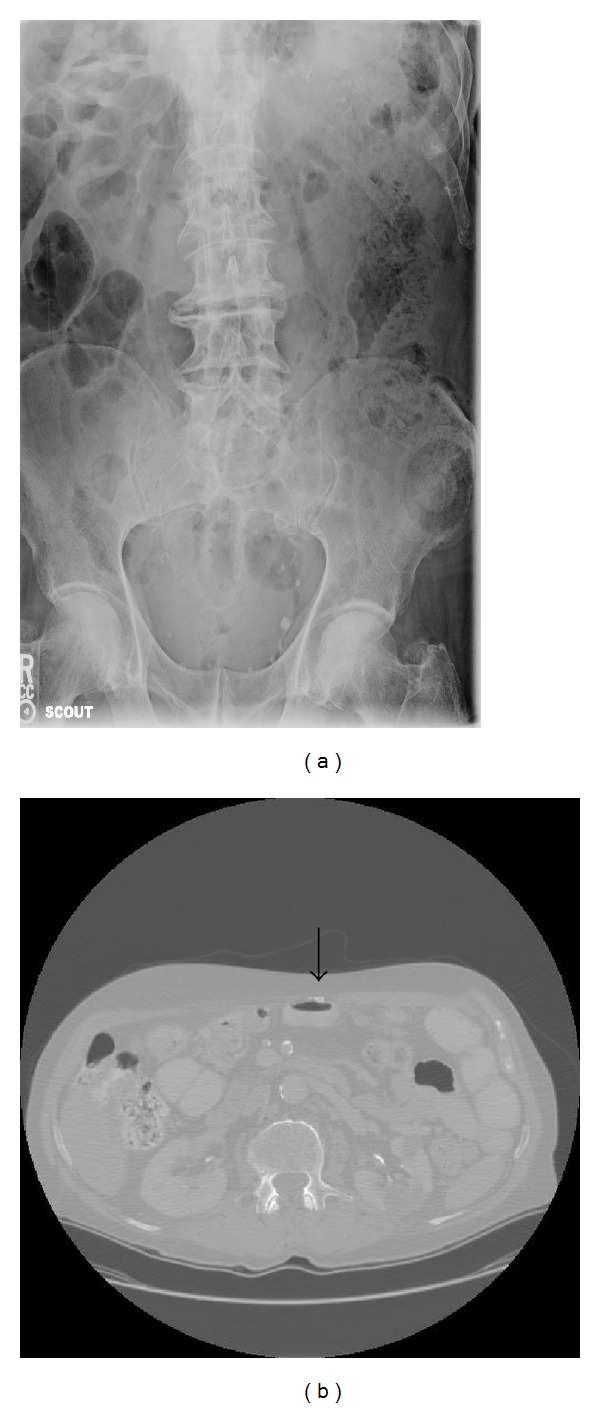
Preoperative abdominal showing (a) no evidence of heterotopic ossification on abdominal plain film. However, there is clear (b) evidence of heterotopic ossification in the lower midline abdominal scar adjacent to bowel wall seen on abdominal CT (computerized tomography) scan. Arrow denotes heterotopic ossification.
